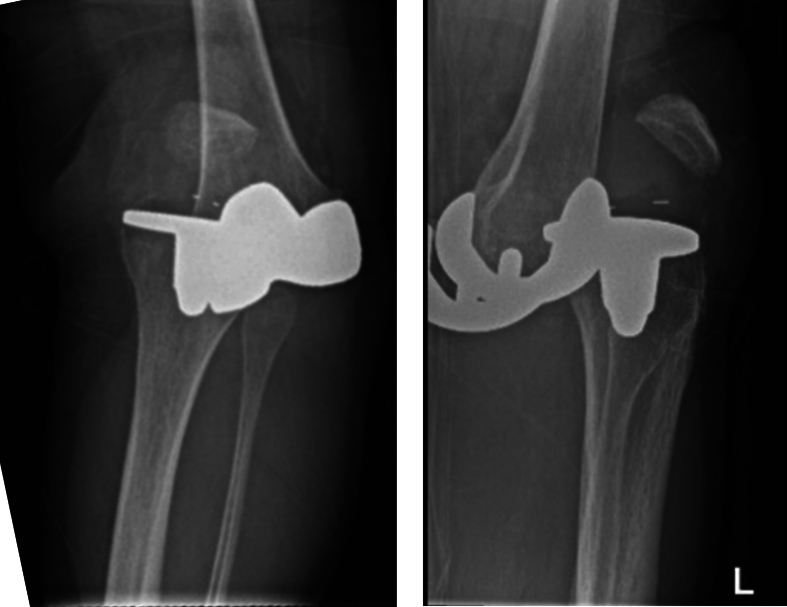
Case 2: conventional radiography images at time of presentation.
